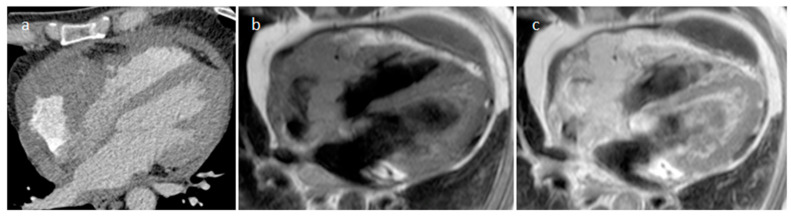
A 37-year-old male patient with a right atrial angiosarcoma at CT (a), on precontrast (b) and postcontrast (c) T1-weighted images.
